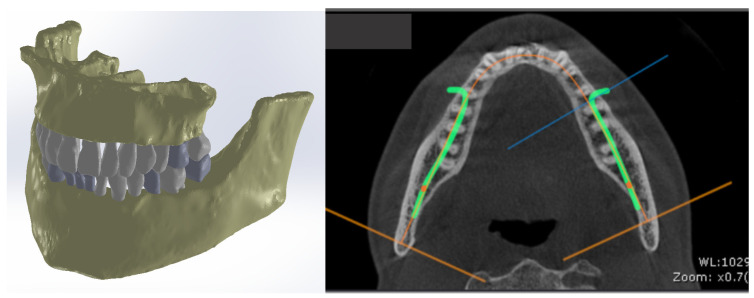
CBCT scan of the patient and the initial model of the orthodontic system.
